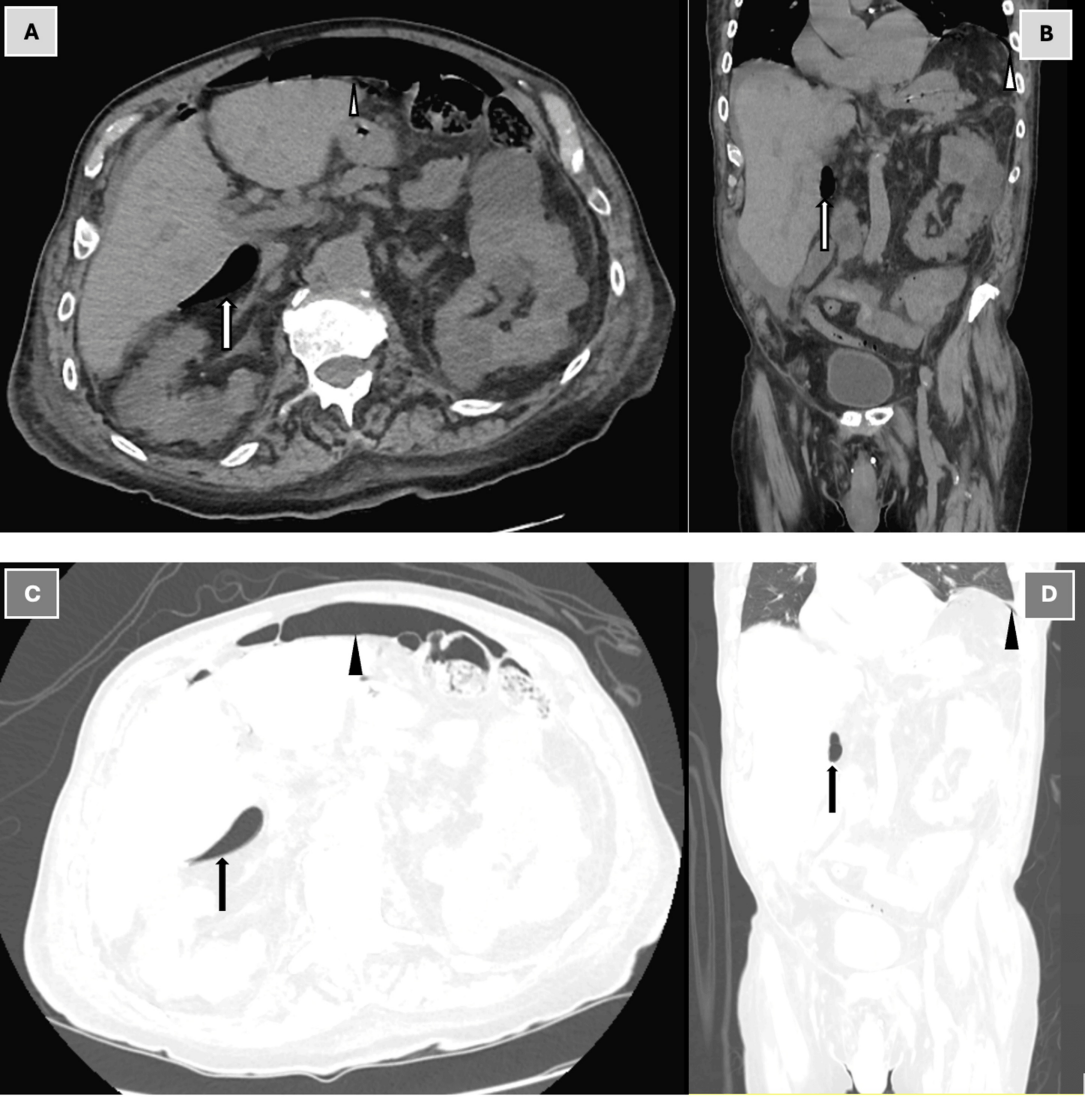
CT of the abdomen and pelvis without intravenous contrast: axial view in soft tissue window (A), coronal view in soft tissue window (B), axial view in lung window (C), and coronal view in lung window (D). CT scan of abdomen and pelvis without contrast showing findings of pneumoperitoneum (triangles) with periduodenal free air in the lesser sac (arrows), suggestive of a perforated duodenal ulcer.
